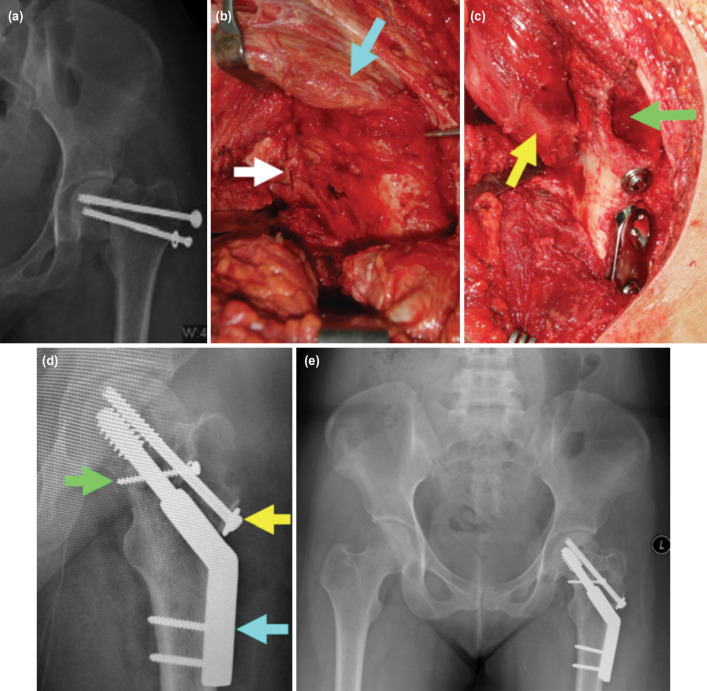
(a) Photos showing the initial screw fixation of case no. 2 with non-union of the femoral neck, varus deformity and neck shortening, (b) open reduction thru Watson Jones approach, anterior one-third of the gluteus medius (blue arrow), reduction of the neck fracture (white arrow), correction of the varus deformity and the neck length, (c) myo-osseous trochanteric flap transferred to the non-union site (yellow arrow), additional bone graft needed was harvested from the trochanter underneath the osteotomised osseous flap (green arrow), (d) post-operative radiograph showing satisfactory reduction and correction maintained with sliding compression screw (blue arrow) and anti-rotational screw (yellow arrow), one cancellous screw (green arrow) secured the myo-osseous flap at the non-union site, (e) radiograph seven years post-surgery with union of the femoral neck without evidence of AVN.
